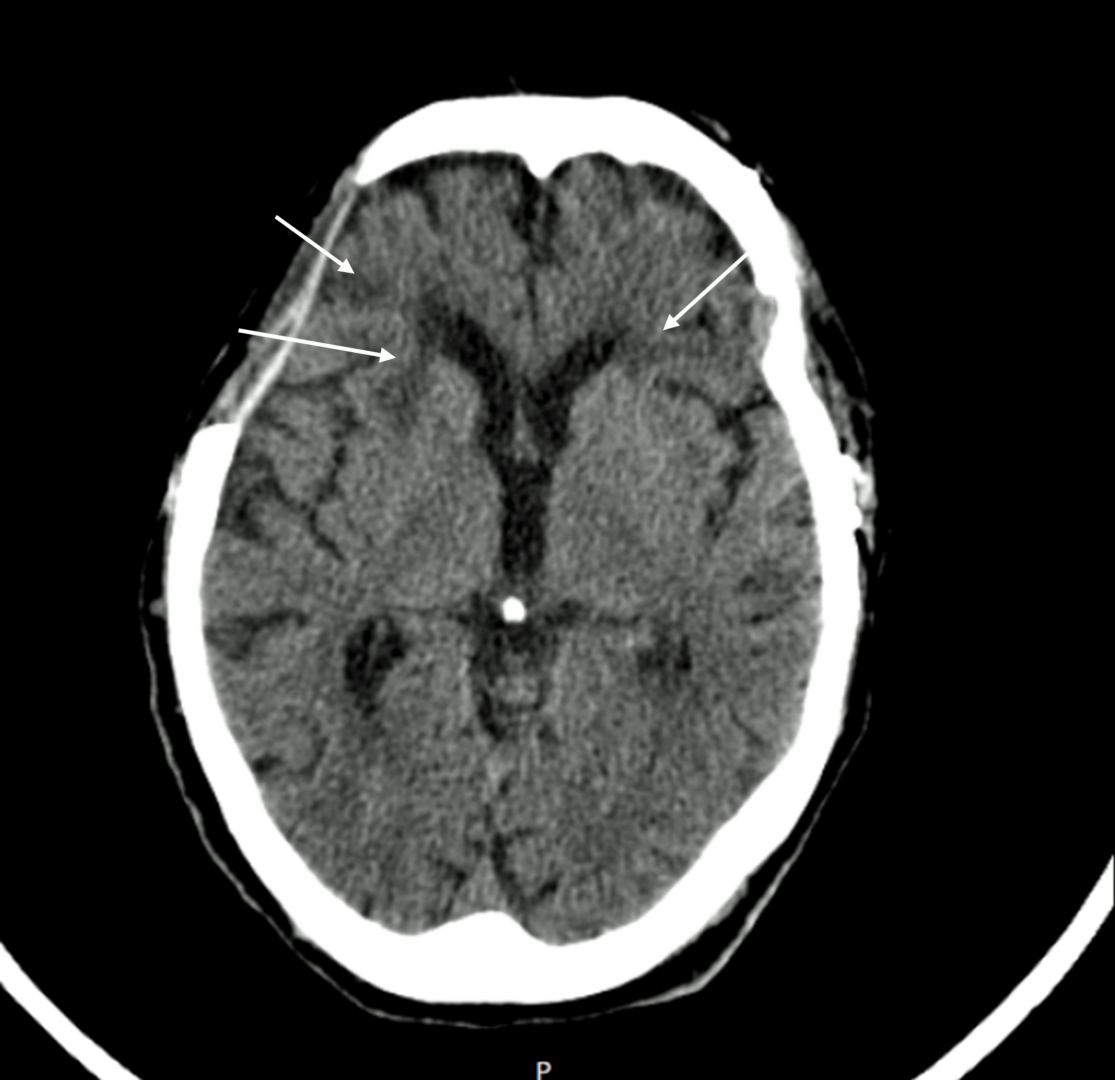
Cerebral CT scan Signs of surgical approach via left fronto-parieto-temporal craniotomy and right fronto-parieto-temporo-pterional craniotomy. Bilateral ischemic cortico-subcortical lesions in the fronto-basal and anterior temporal and periventricular regions.
